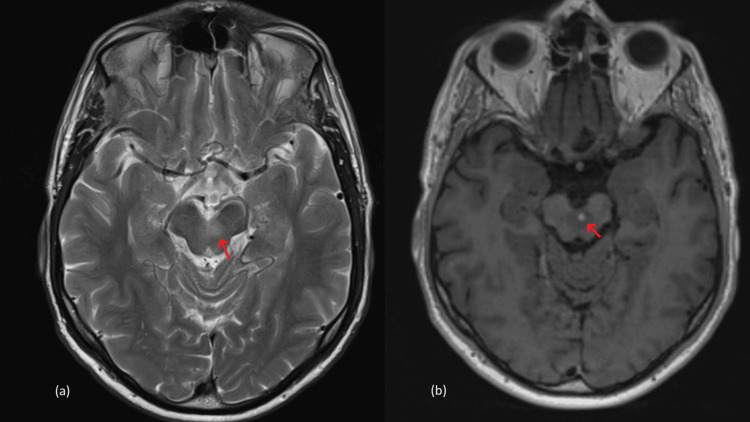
MRI IAM images (a) T2 weighted axial sequence, (b) T1 space post contrast axial sequence. Internal auditory meatus (IAM) magnetic resonance image (MRI). (a) Axial image through the ventral midbrain showing left oedema. (b) Axial MRI selected section through the ventral midbrain showing an enhancing nodule indicating left rhombencephalitis. Both areas of interest have been circled.
